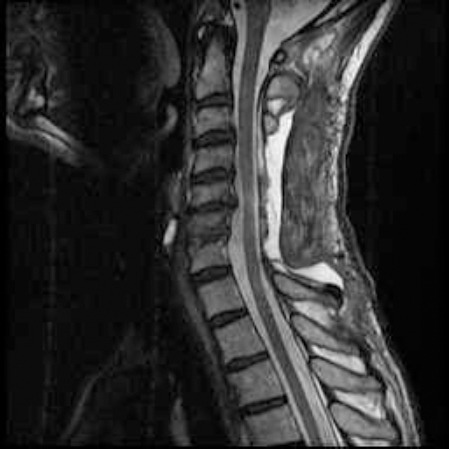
There is no arachnoid cyst and the spinal cord anterior subarachnoid space has expanded in postoperative T2 cervical MRI sagittal section*.
